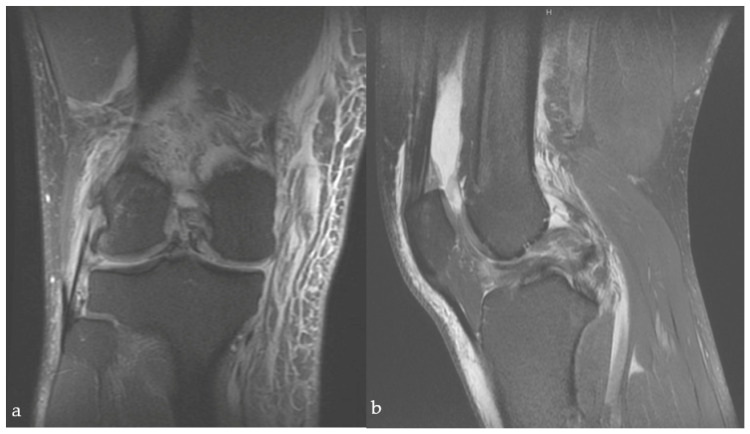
Sagittal (a) and frontal (b) view of a T2-weighted MRI scan of a right knee 2 days after KD type IV with disruption of both cruciate and bilateral bucket handle tear.
